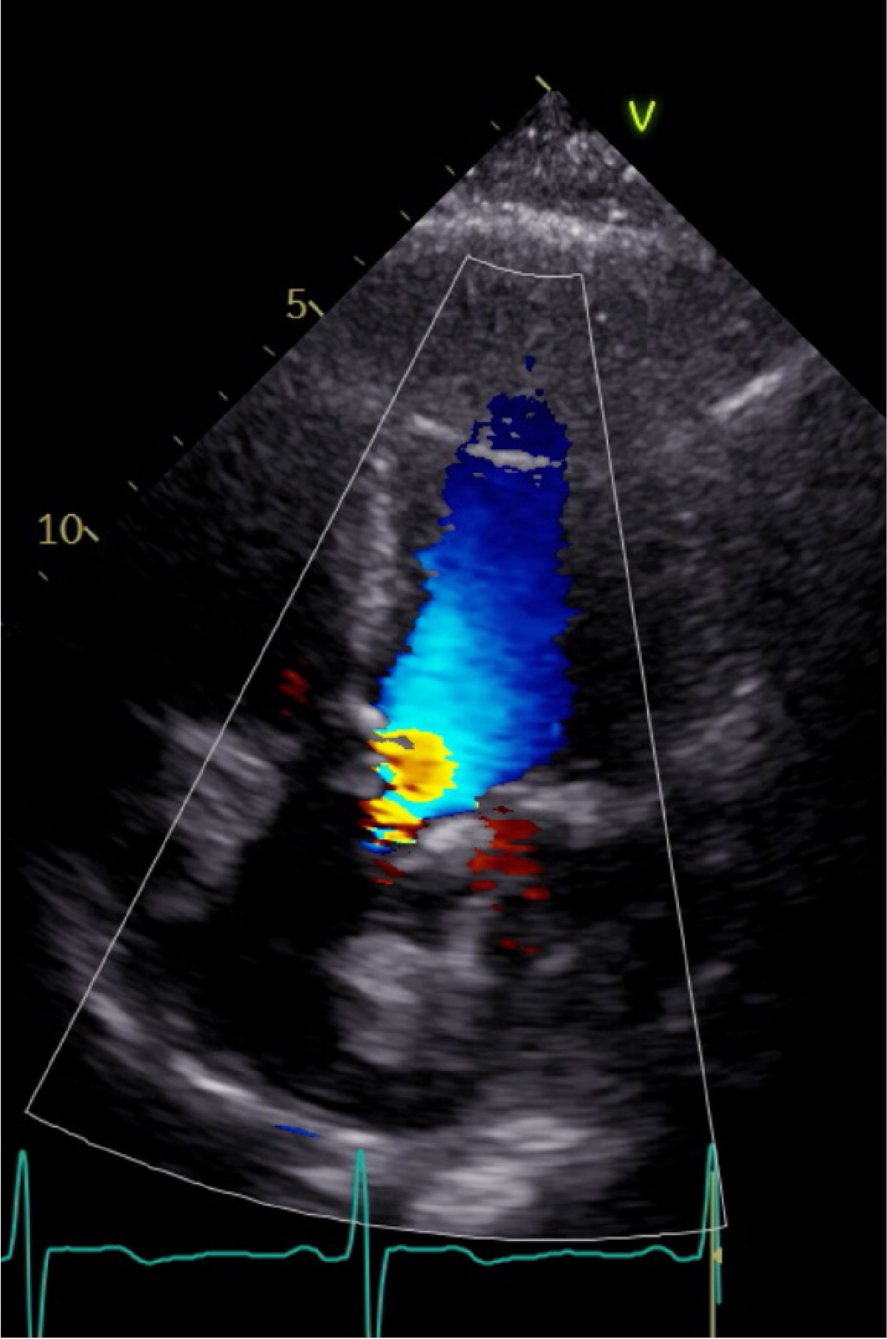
Preoperative echocardiography. Apical long-axis view showing mosaic flow and accelerated flow across the aortic valve
